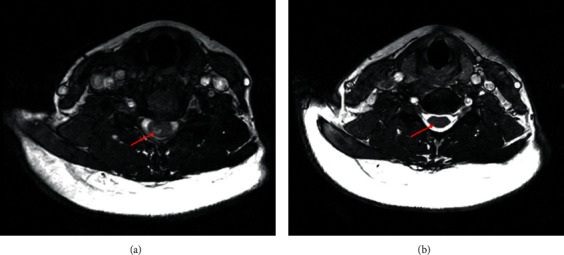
MRI of the cervical spinal cord-axial T2-weighed sequences ((a), (b)). (a) Sequence from February 2019 shows an abnormal signal intensity that involves the center of the cord and minimally extends to the cord's periphery. (b) Sequence from June 2020 shows an interval decrease in both swelling and hyperintensity.
